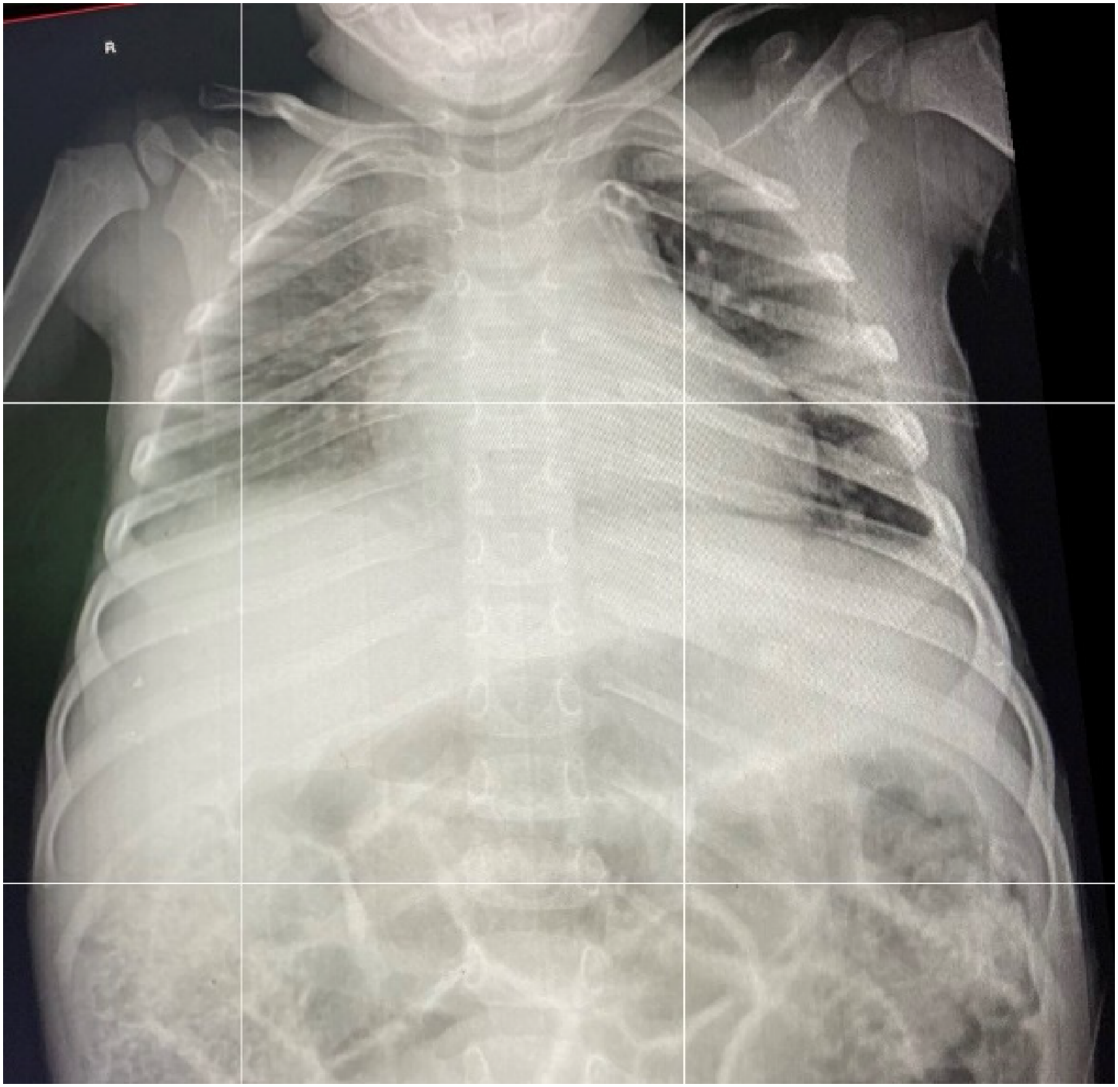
An AP chest x-ray taken 48 h postoperation prior to the removal of the chest tube.
